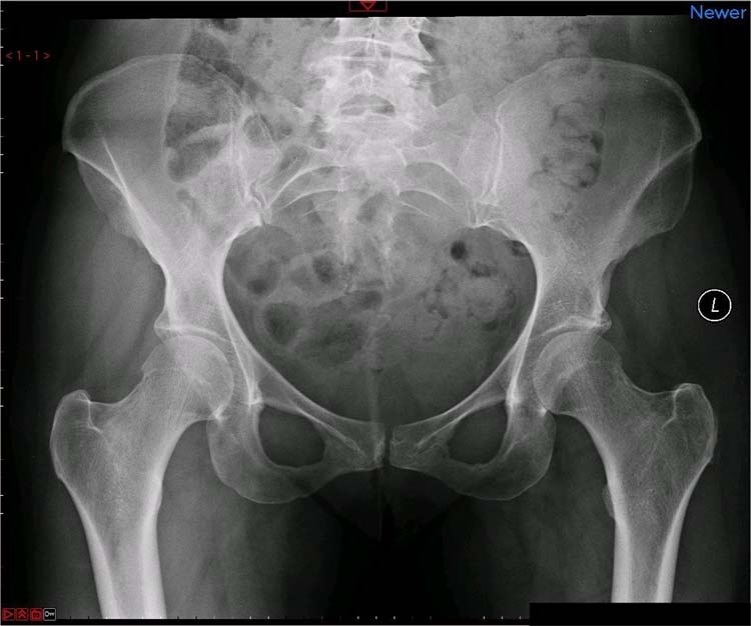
Preoperative X-ray of the pelvis: preoperative X-ray of the pelvis, performed in March 2014, showing no implants yet in place.
